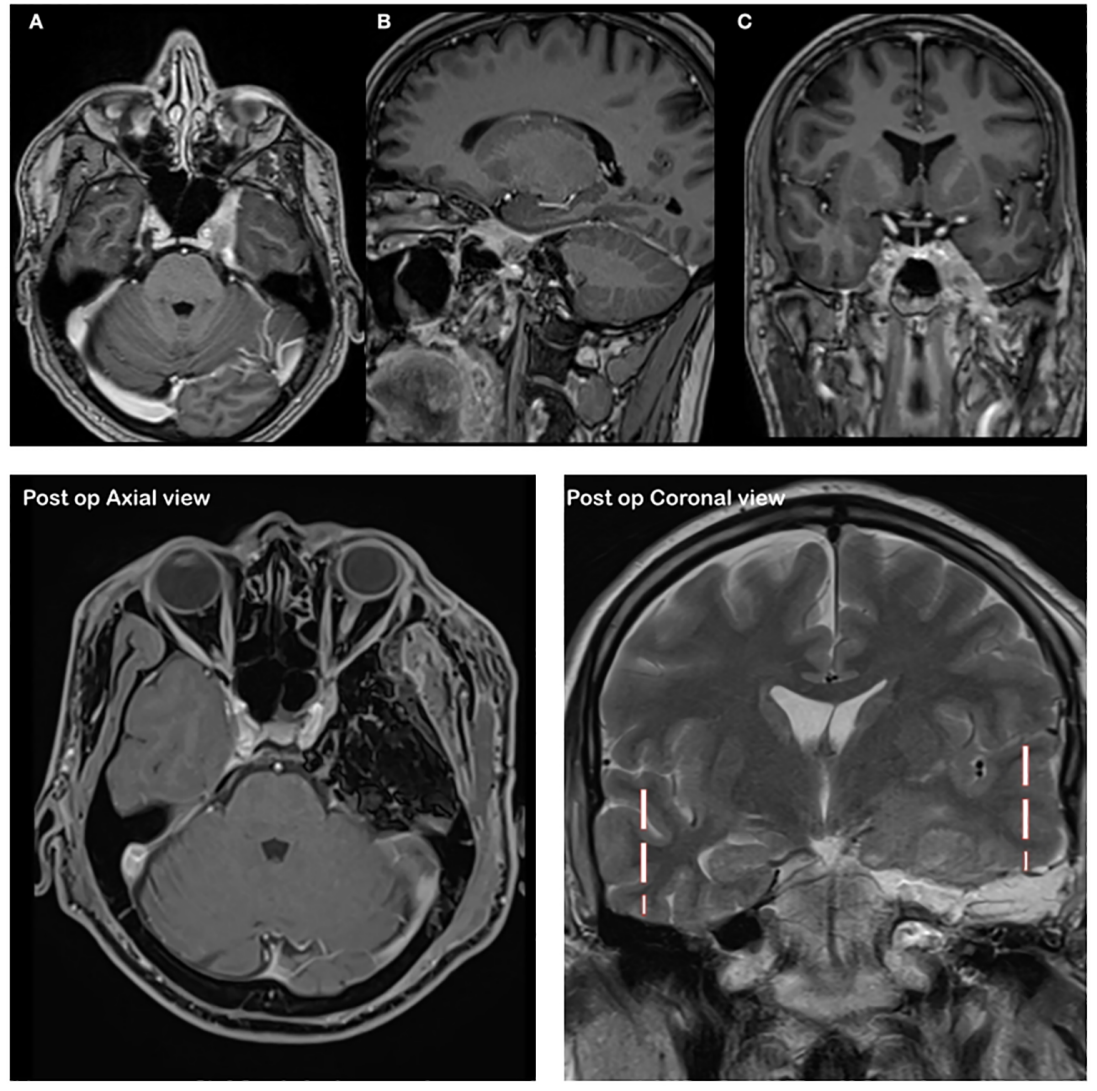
Preoperative axial (a), sagittal (b) and coronal (c) T1-weighted post-gadolinium MRI demonstrating enhancing tumor extension along the mandibular division of the trigeminal nerve(V_3_) into Meckel’s cave and the cavernous sinus, with encasement of the internal carotid artery and evidence of perineural spread. Postoperative contrast-enhanced, fat-suppressed T1-weighted axial MRI demonstrating complete tumor resection with the surgical cavity filled by fat graft. Coronal MRI show natural elevation of the temporal lobe from the skull base, with excellent preservation of temporal lobe integrity.
